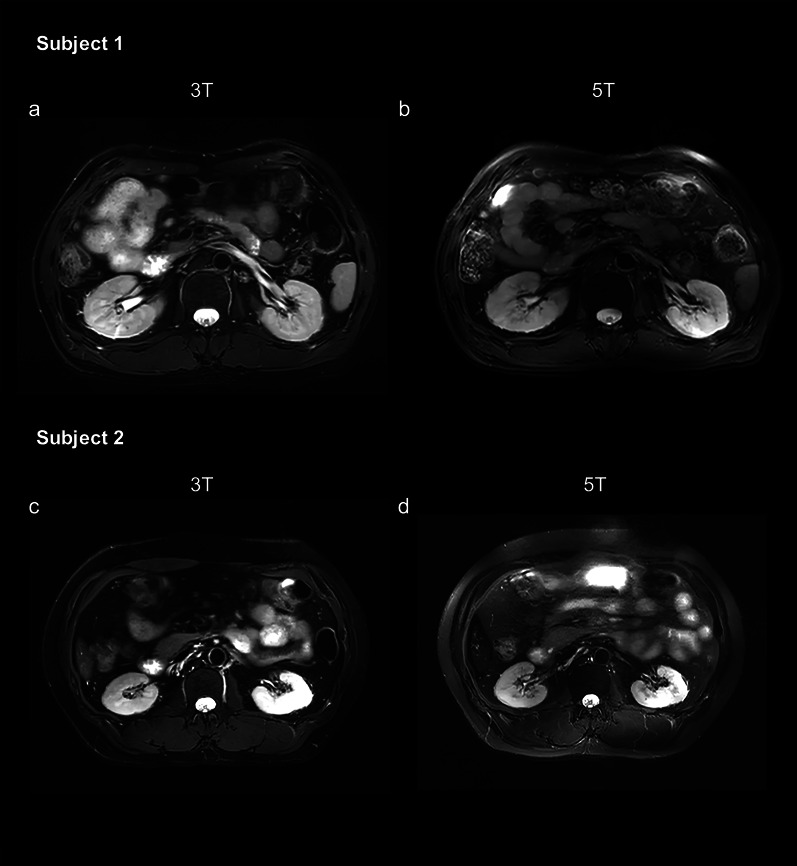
Axial, T2-weighted fast spin echo (FSE) sequence at 3 T (a and c) and 5 T (b and d) from subject 1 (49-year-old male) and subject 2 (40-year-old male). For subject 2, T2-weighted FSE at 5 T exhibited a higher level of artifacts compared to the 3 T examination
